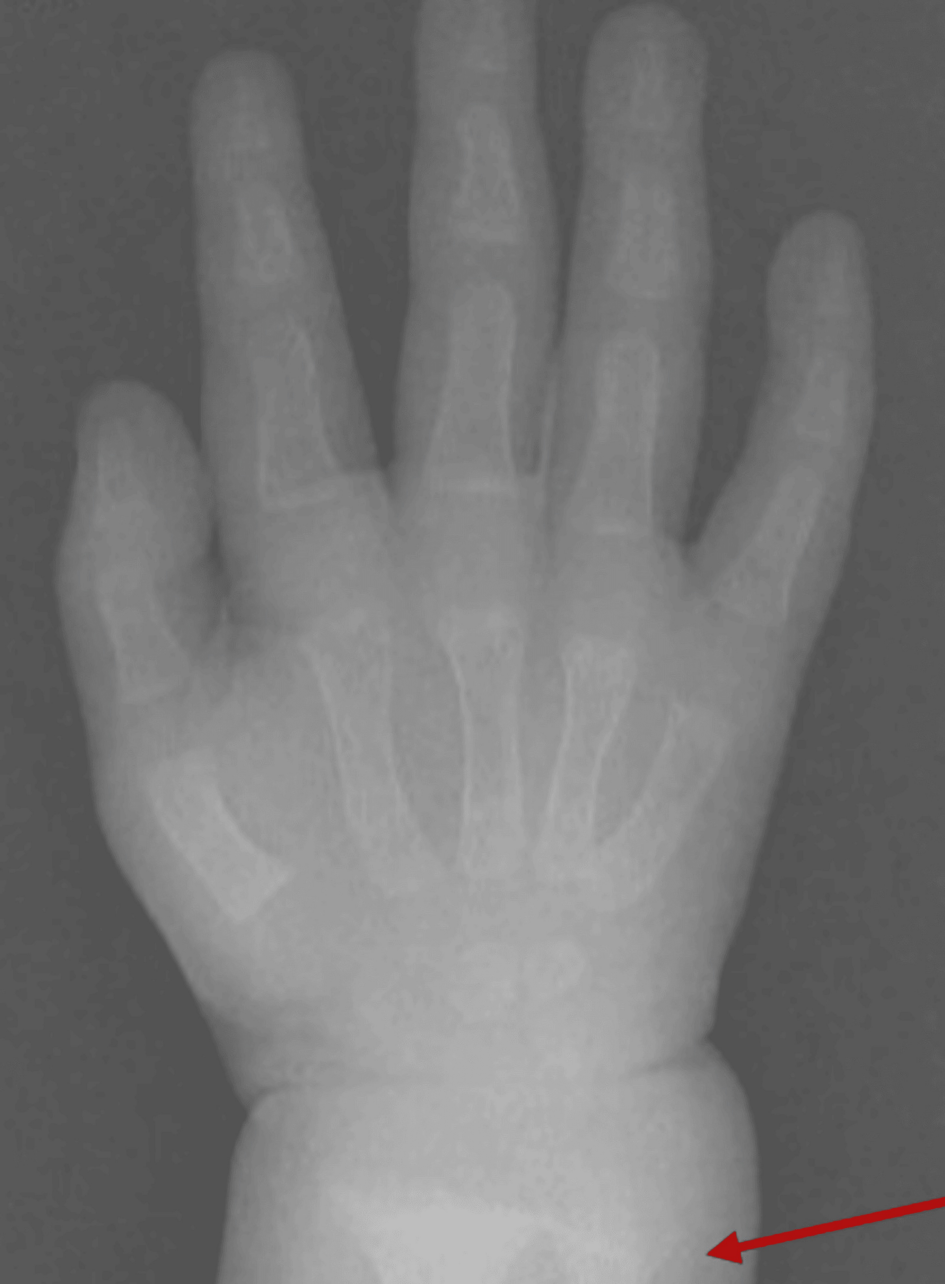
Wrist X-ray showing metaphyseal cupping and fraying (arrow), consistent with active rickets
